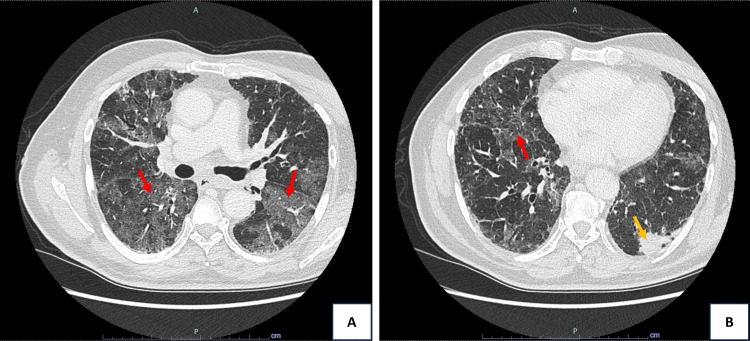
Axial chest CT scans obtained after the second cycle of durvalumab (three months after radiotherapy and two months after initiation of immune checkpoint inhibitor therapy) (A) and (B) show multifocal ground-glass opacities involving all lobes (bilateral patchy inflammatory lung infiltrates (red arrows)), consistent with pneumonitis. A neoformative lesion in the posterobasal segment of the left lower lobe shows no evidence of progression (yellow arrow).
